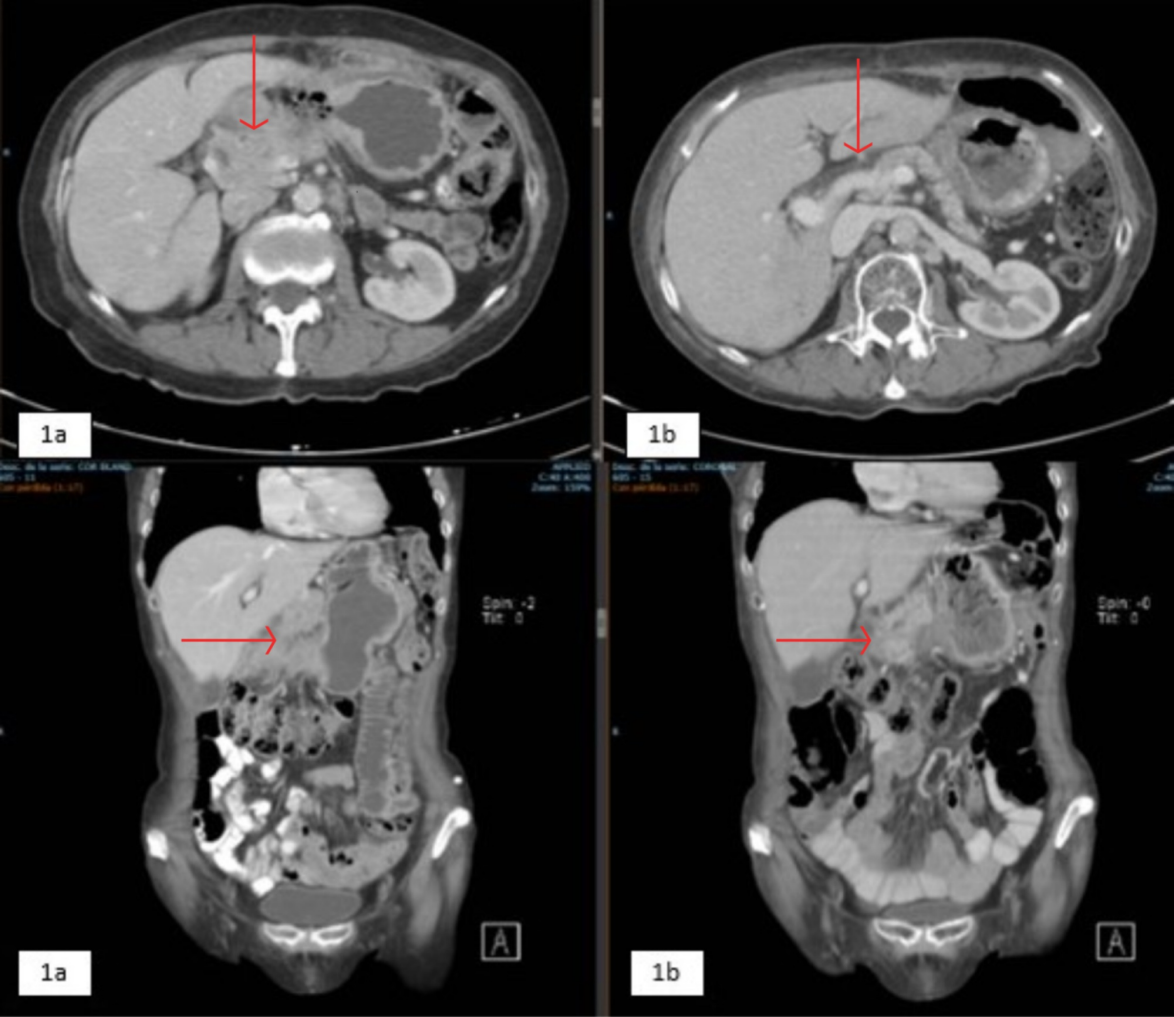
Computed tomography of the abdomen and pelvis 1a. Thickening of the neoplastic aspect in the antropyloric region of the stomach with signs of extension beyond the serosa, the involvement of the gastrocolic ligament, with suspicious retropancreatic adenomegaly, which conditions the complete occlusion of the pylorus, with gastric bypass via gastrojejunal anastomosis when compared to the previous study. 1b. Post-surgical changes due to gastric bypass and functioning intestinal anastomosis; an almost-complete resolution of the thickening of the antropyloric region walls, persisting alteration of regional fat, with no evidence of nodules. Resolution of the retroperitoneal paraaortic and gastrohepatic ligament nodal involvement.
